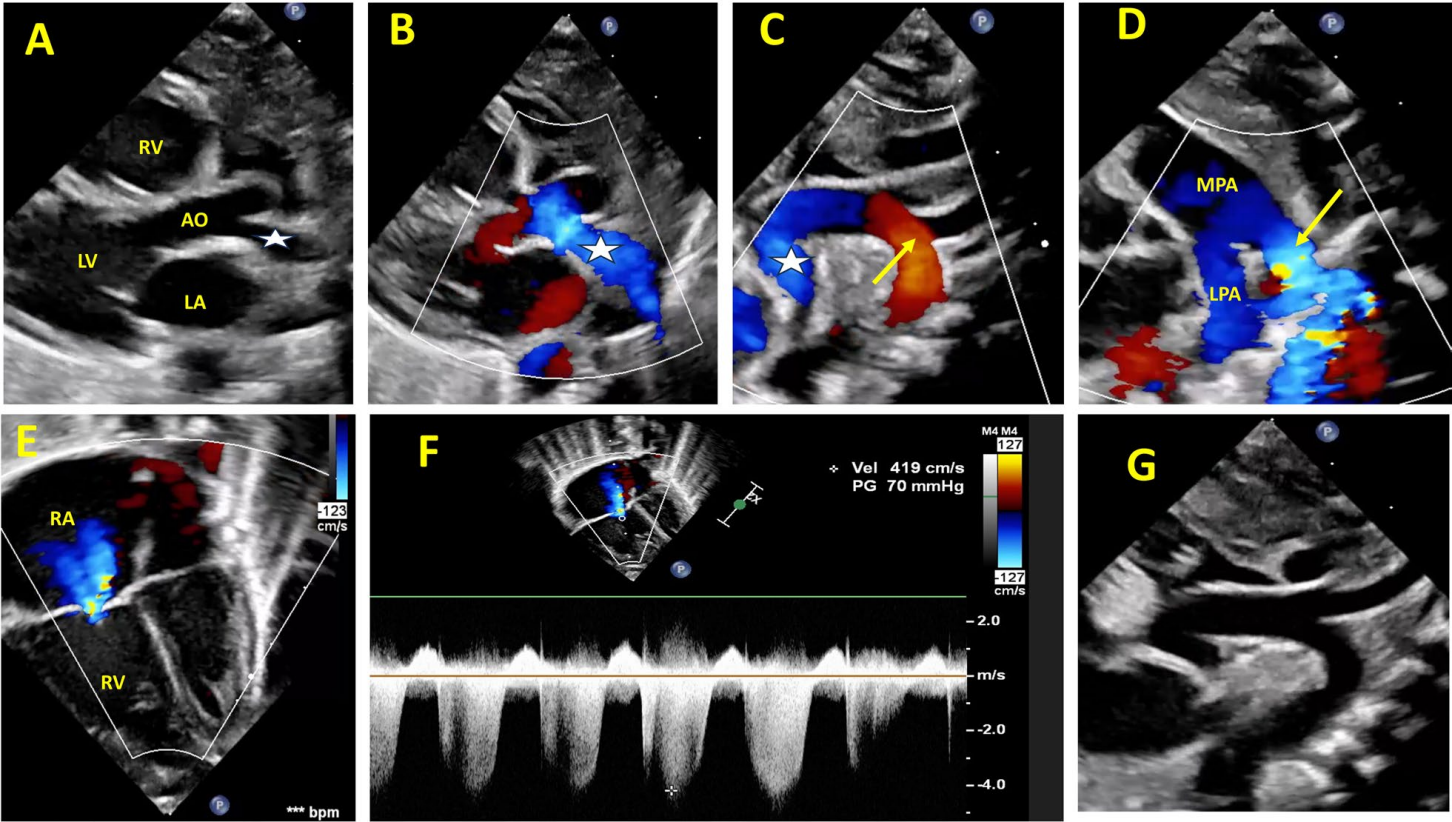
A 2D image of parasternal long axis view showing the RPA arising from the ascending aorta (white asterisk). B Color Doppler of parasternal long axis view showing the RPA (white asterisk). C: Suprasternal long axis view showing RPA (white asterisk) and the retrograde flow in the aortic arch (yellow arrow). D The parasternal short-axis view shows the right-to-left PDA flow (yellow arrow). E An apical four-chamber view shows moderate tricuspid regurgitation (TR) with dilated RA and RV F Continuous wave Doppler at TR showing a TR gradient of 70 mmHg (indicating severe pulmonary hypertension). G Suprasternal long axis view showing the aortic arch and the proximal descending aorta with no coarctation. Ao: aorta: LA: left atrium, LPA: left pulmonary artery, LV: left ventricle, MPA: mean pulmonary artery, PDA: patent ductus arteriosus, RA: right atrium, RPA: right pulmonary artery, RV: right ventricle, TR: tricuspid regurgitation
